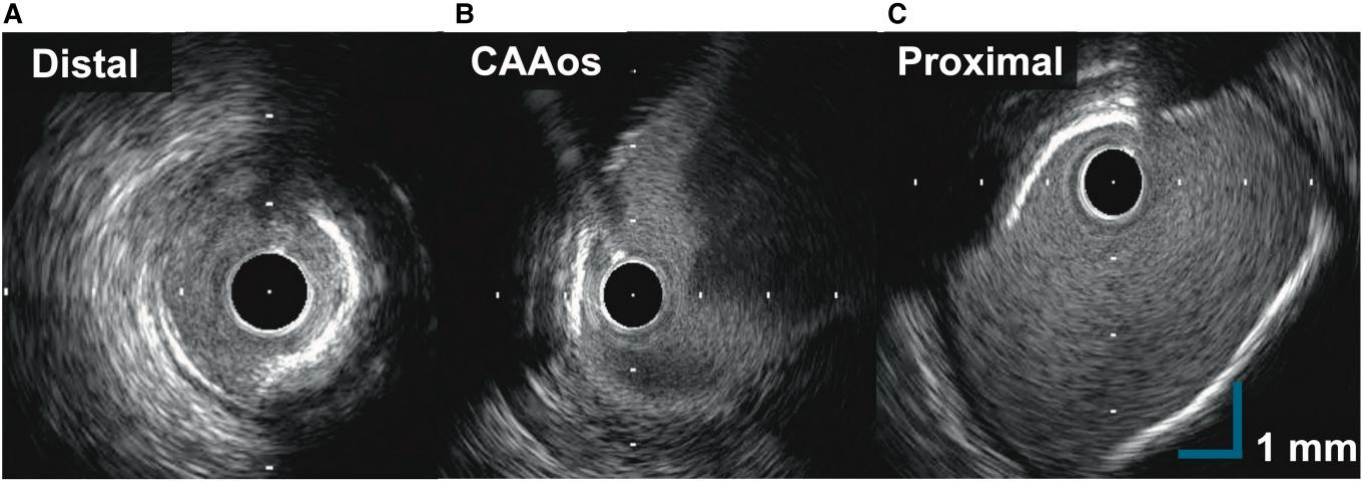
Intravascular ultrasound (IVUS) imaging of the aneurysm. IVUS image delineated the aneurysm neck and the adjacent LAD vessel wall. (*A*) IVUS of the distal LAD; (*B*) IVUS showing the aneurysm ostium from within the LAD; (*C*) IVUS of the proximal LAD. These images delineate the aneurysm neck and the adjacent LAD vessel wall. The entry point from the LAD into the aneurysm and vessel wall integrity is clearly visualized. The entry point from the LAD into the aneurysm and vessel wall integrity is clearly visualized.
